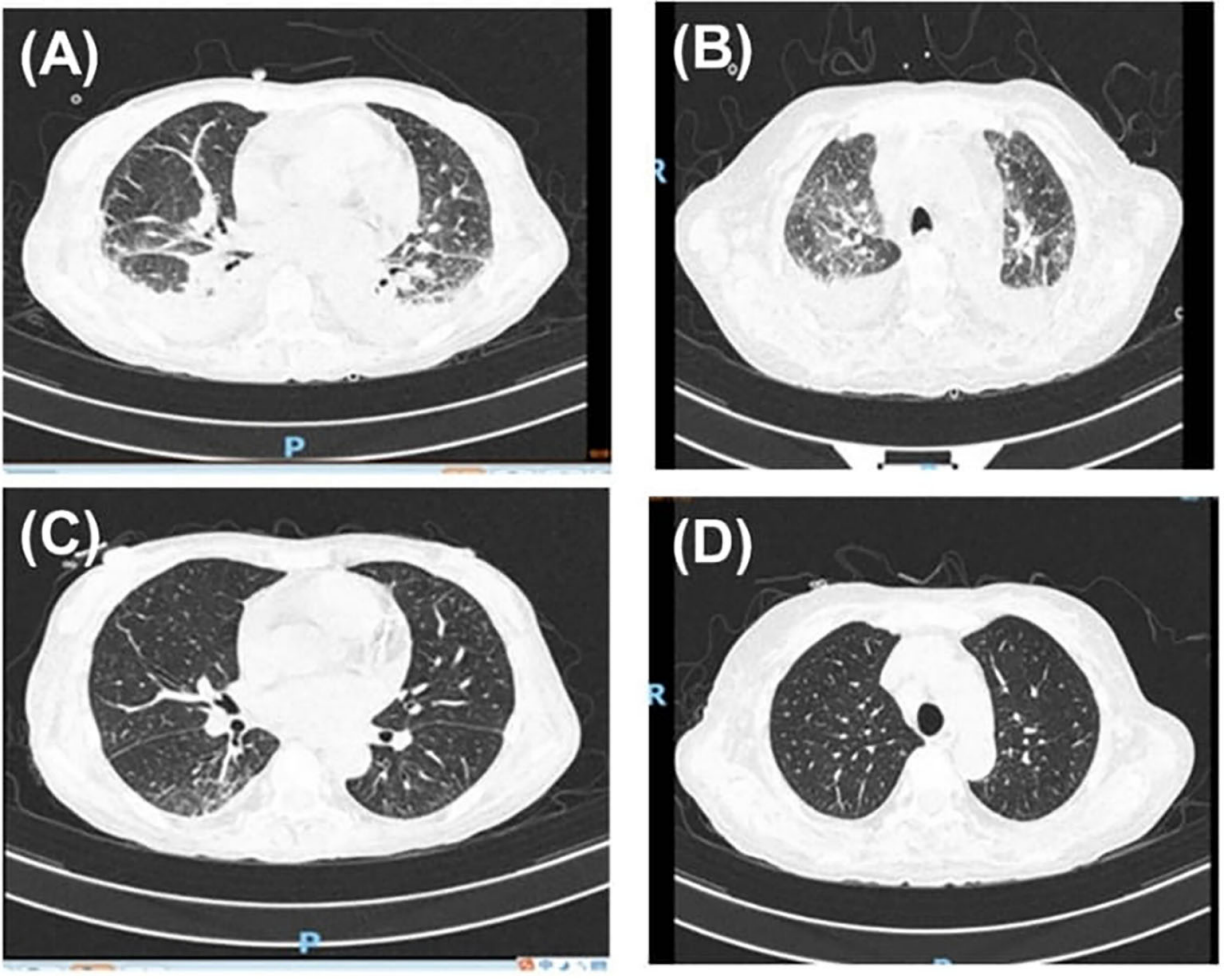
Radiological comparison of chest and brain before and after treatment. (A, B) Chest CT obtained in March 2024, prior to treatment showing bilateral pulmonary infiltrates with multifocal ground-glass opacities. (C, D) Chest CT obtained in April 2024, after treatment demonstrating interval improvement of the pulmonary infiltrates.
